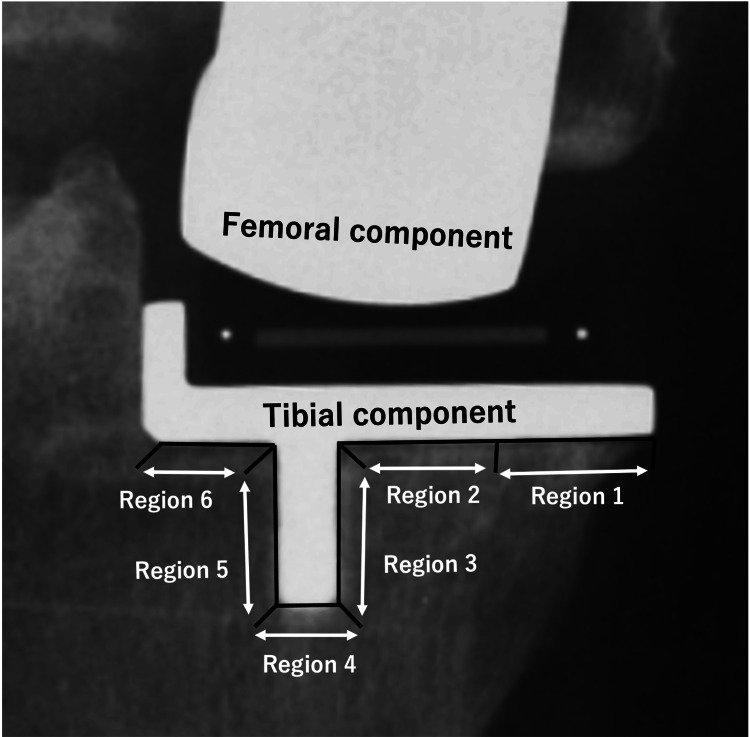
Radiograph coronal view of tibial component Radiograph showing the six regions for the distribution of radiolucent lines beneath the tibial component of the Oxford UKA UKA - unicompartmental knee arthroplasty
